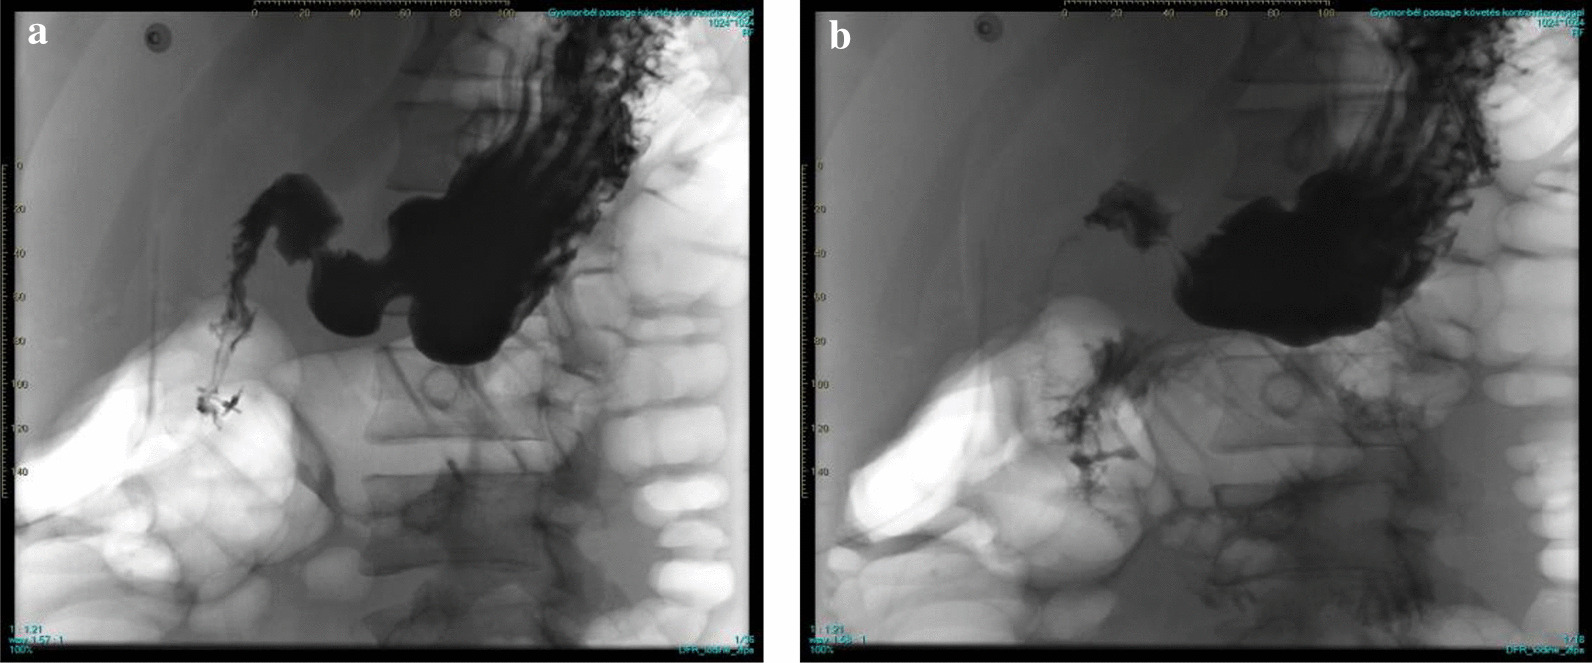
Contrast radiography of the upper gastrointestinal tract 3 days after the surgery. Early contrast radiography of the stomach (a). Late contrast radiography with contrast agent diffusion next to the clipped duodenum (b)
